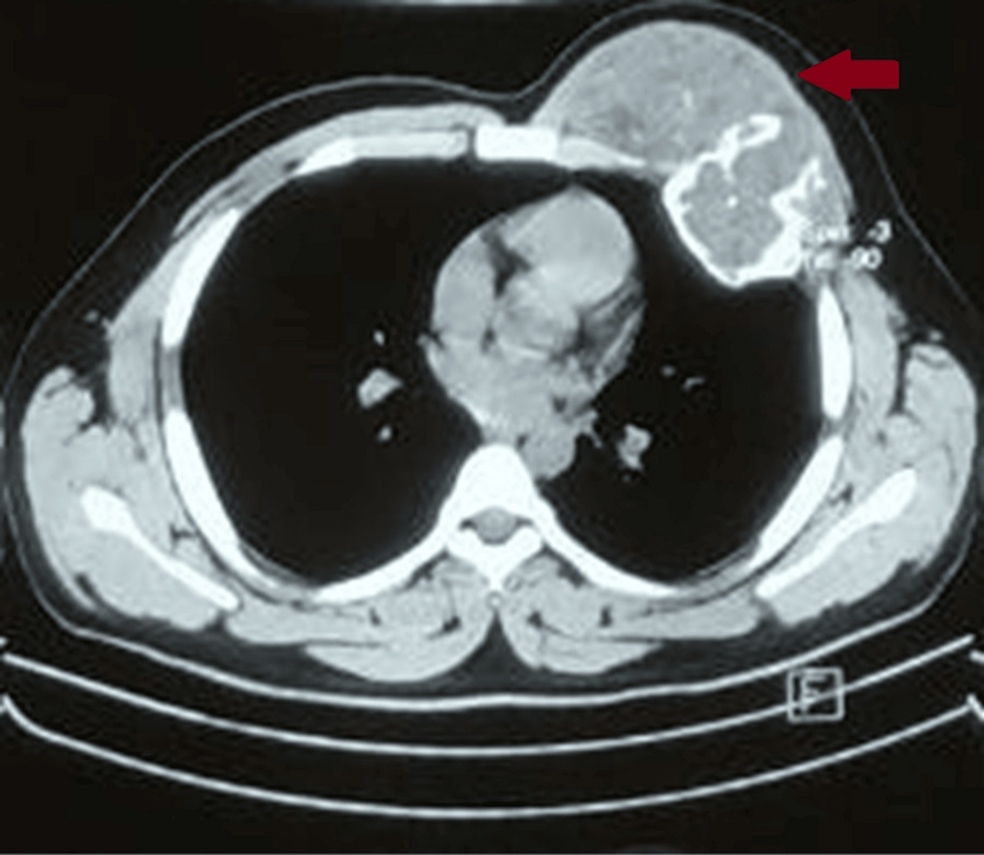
CT scan of the chest CT scan showing growth from the left chest wall (indicated by red arrow).
